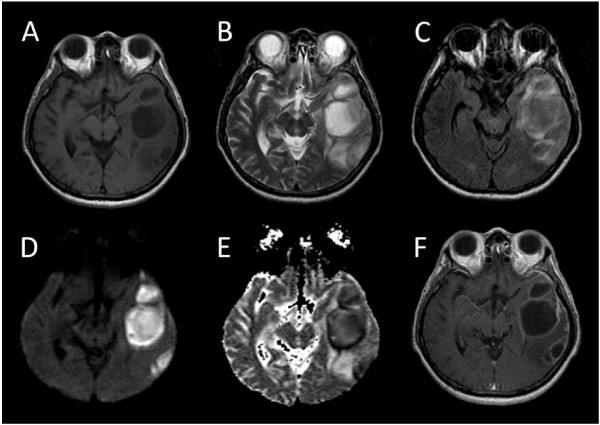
(A) Pre-contrast axial T1-weighted MR revealed multiple left temporal lesions with hypo-intense central cavity and moderately hypo-intense surrounding edema.(B) T2-weighted and (C) fluid attenuated inversion recovery (FLAIR) axial MR revealed multiple hyper-intense lesions and surrounding hyper-intense edema. (D) Diffusion-weighted MR demonstrated high signal intensity, with (E) a corresponding reduction in the apparent diffusion coefficient. This was directly related to the cellularity and viscosity of the pus contained within the abscess cavities. In contrast, tumors with central necrosis had an appearance of marked hypo-intensity on diffusion-weighted images and hyper-intensity on the apparent coefficient map. (F) Post-gadolinium T1-weighted scan showed thick ring enhancement and increased enhancement of the adjacent dura
